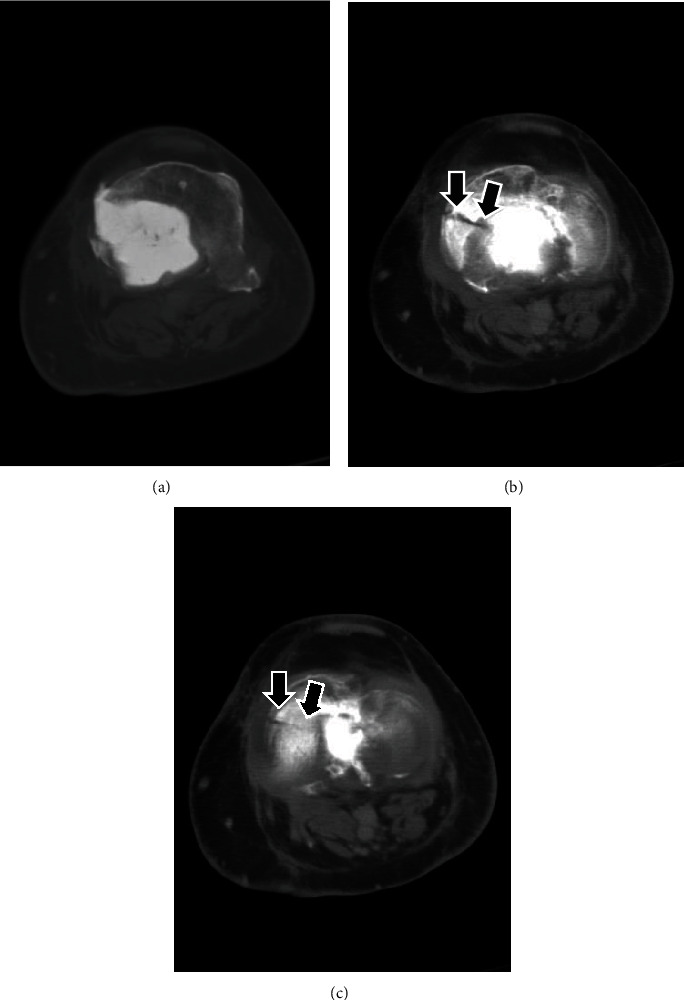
Axial CT-scan at 14 months postoperatively, moving from distal (a) to proximal (c). The PMMA bone cement is still contained; however, the proximal tibia of the patient sustained a pathological fracture (black arrows) after a fall.
